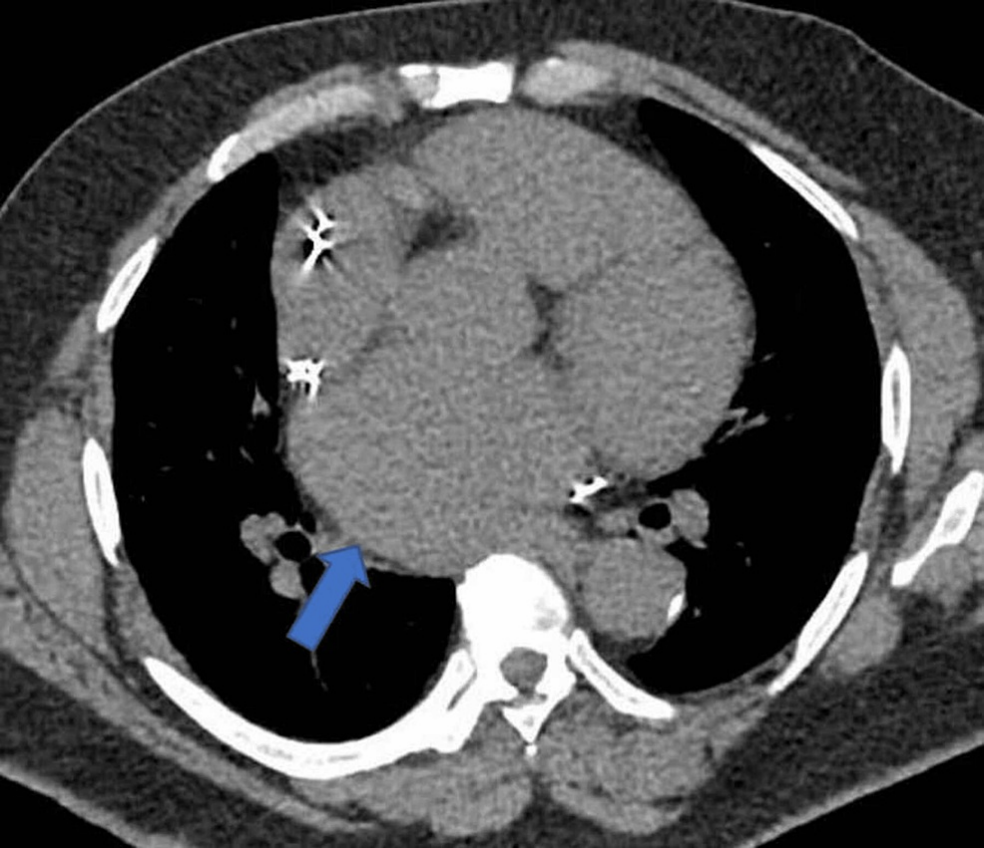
CTPA showing enlarged left atrium (blue arrow) CTPA: computed tomography pulmonary angiogram
